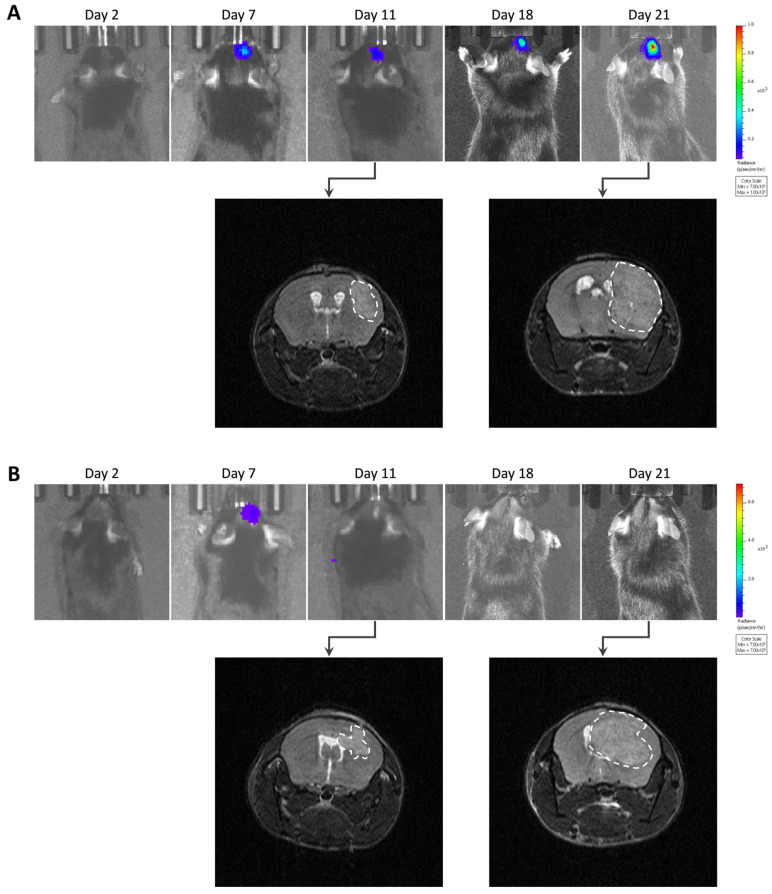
Images of GL261-luc-GFP tumor growth by bioluminescence imaging (BLI) and magnetic resonance imaging (MRI). (A) Representative images of a tumor’s growth with consistent data observed with both BLI and MRI. (B) Representative images of another case where BLI showed signal decrease over time, suggesting a regression, while MRI showed that the tumor was actually growing.
